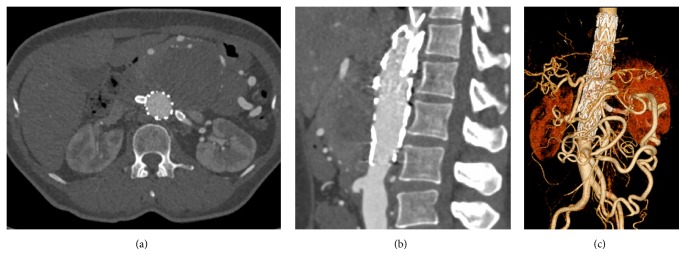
CTA: axial view, no endoleak (a). Sagittal view: stent placed superior to inferior mesenteric artery orifice (b). Reconstruction view: adequate flow from the inferior mesenteric artery to supply the celiac artery and superior mesenteric artery.
